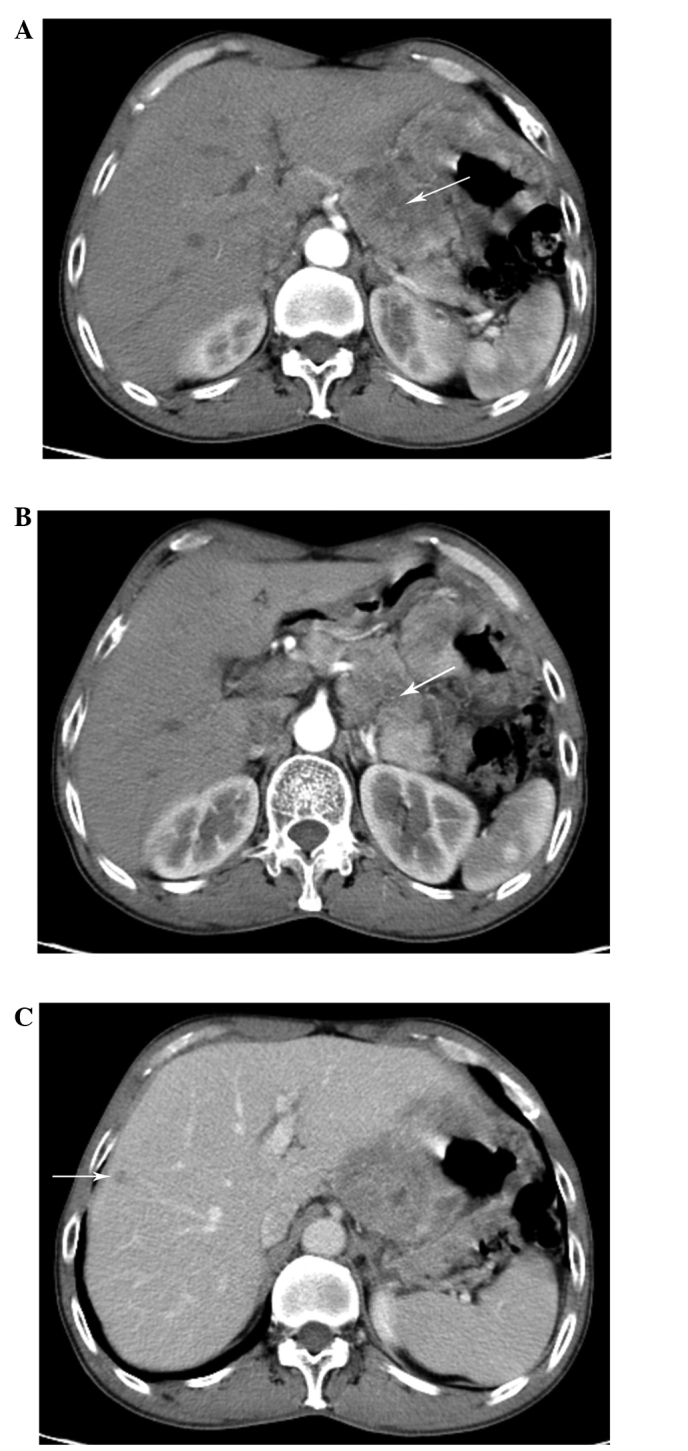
Enhanced computed tomography prior to the neoadjuvant chemotherapy. (A) A mass (arrow) in the gastric wall. (B) The mass (arrow) invading the liver and pancreas. (C) A nodular shadow (arrow) of low density, with mild enhancement.
